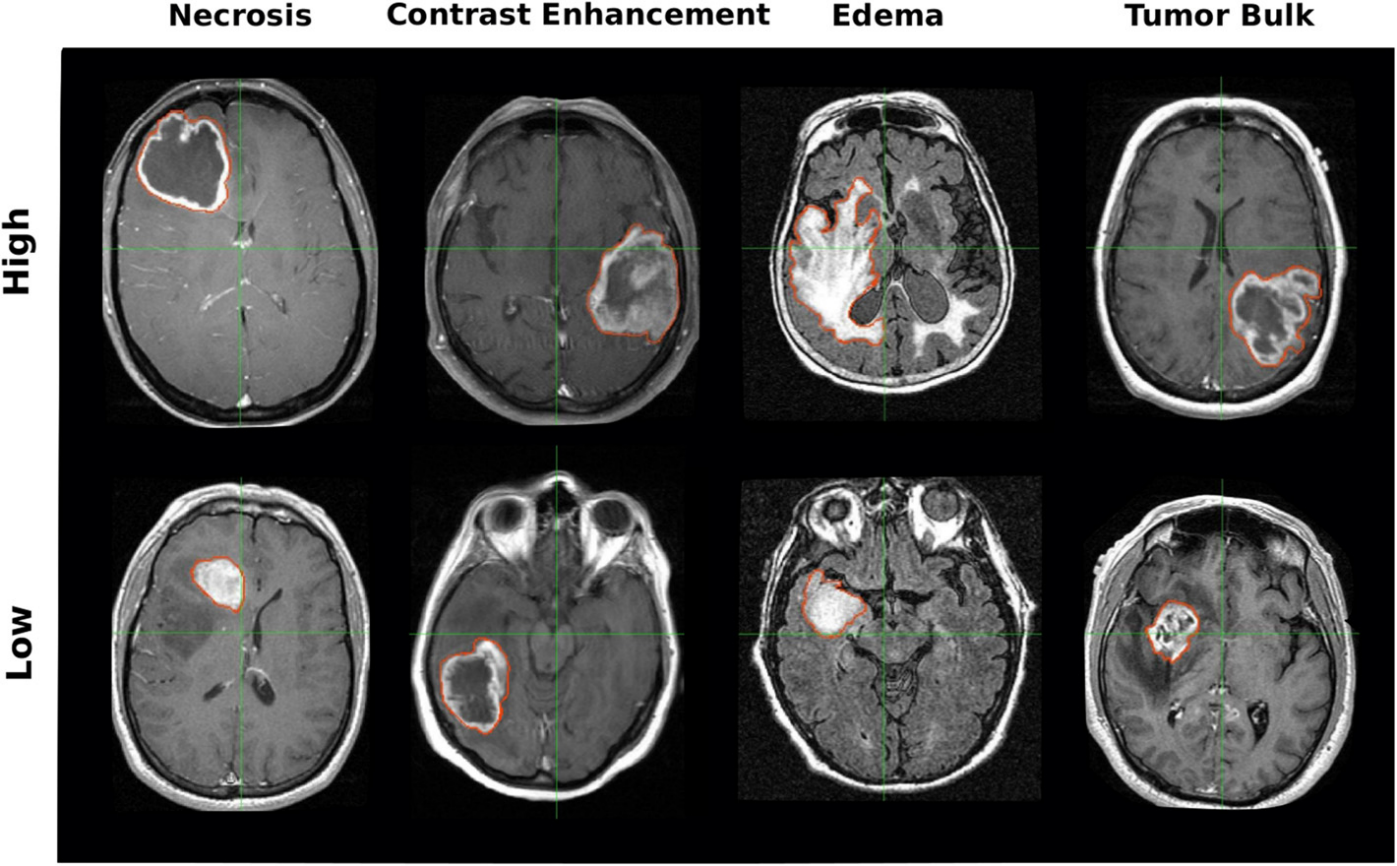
Examples of volumetric tumor phenotype features. Glioblastoma (GBM) tumors show strong phenotypic differences, which can be objectively quantified with volumetrics. This figure shows examples of GBM tumors exhibiting high (top) and low (bottom) volumetric feature values for Necrosis, Contrast Enhancement, Edema, and Tumor Bulk (columns) as they appear on T1 weighted (columns 1,2, and 4) or T2-FLAIR (column 3) magnetic resonance images for different patients
